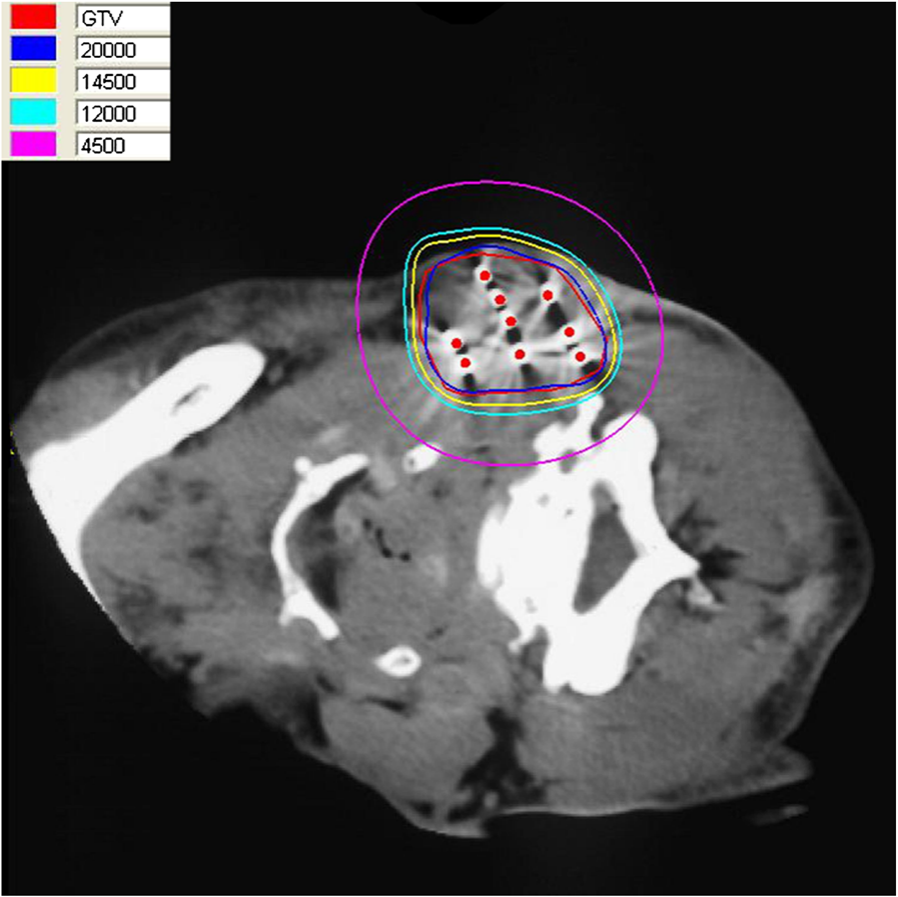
The isodose curve distribution of tumor after seed implantation from CT scan. The inner red curve represents tumor. The ellipses are iso-dose lines of 200, 145, 120 and 45 Gy from inside, respectively.
